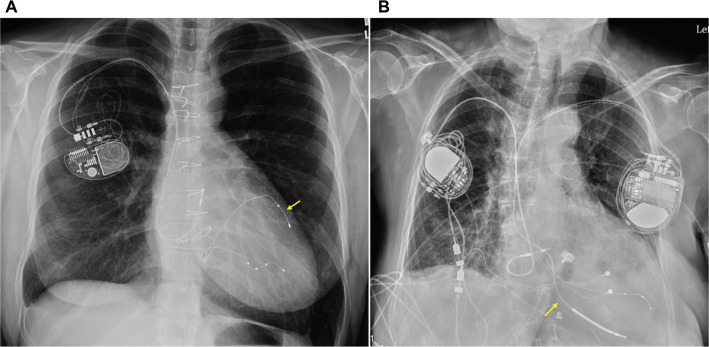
A: Frontal chest radiograph of a patient with a biventricular permanent pacemaker with two ventricular leads in the coronary sinus system. A bipolar lead (arrow) is in the anterior interventricular vein and a quadripolar lead is in a posterolateral branch. B: Frontal chest radiograph of a biventricular implantable cardioverter-defibrillator system with an implantable cardioverter-defibrillator lead in the middle cardiac vein (arrow) connected to a left-sided generator. Of note, there is a non-functional right-sided permanent pacemaker system that includes an epicardial ventricular lead.
